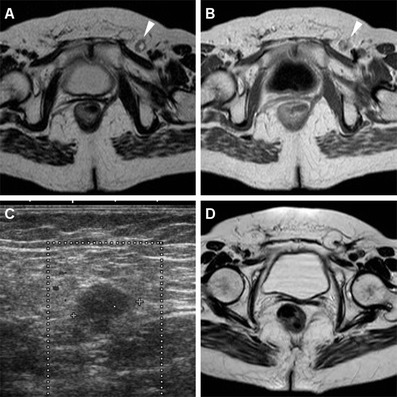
A 65-year-old woman with history of previously treated small SCAC 3 years earlier. Axial T2- (a) and post-contrast T1-weighted (b) images show roundish 1-cm left inguinal node (*arrowheads*) with internal fluid-like necrosis and inhomogeneous enhancement, confirmed by ultrasound (c) as hypoechoic with loss of normal nodal structure. Surgical exeresis (postoperative status as seen in d, follow-up MRI) confirmed metastatic node from SCAC
